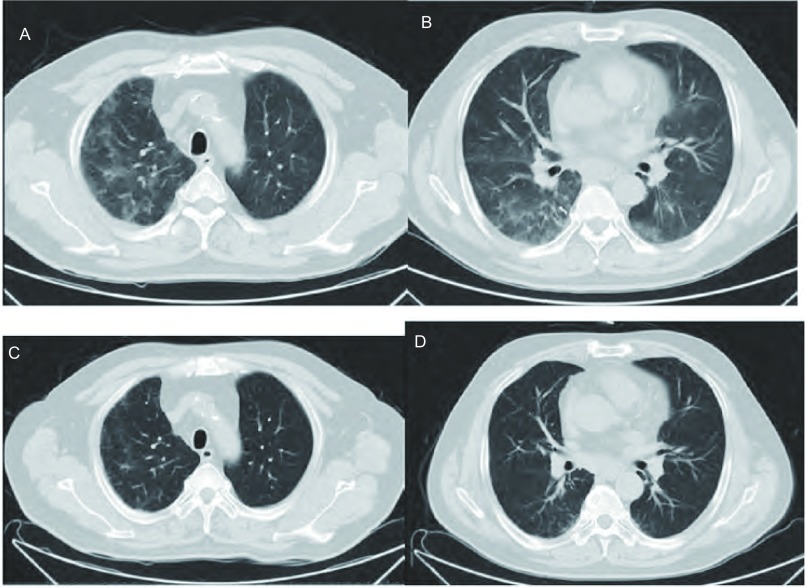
胸部CT检查结果。A、B：胸部CT示双肺间质性渗出，肺泡间隔增厚，多发斑片状、片状磨玻璃密度影；C、D：胸部CT示双肺间质性炎症较前明显好转。 Chest computed tomography findings on admission. Chest computed tomography (A, B) revealed diffuse interstitial lesions with thickening of the alveolar septa and multiple patchy, flake grinding glass opacity. Chest computed tomography (C, D) findings were substantially improved after initiating steroid pulse therapy.
